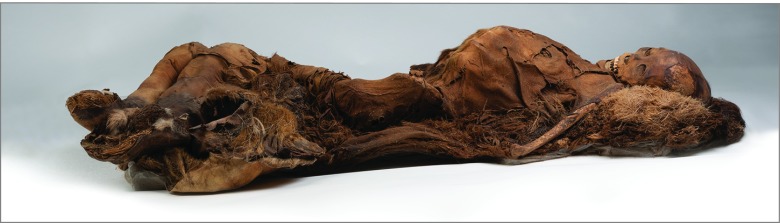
Adult Inuit Mummified Individual Who Was Scanned With Computed Tomography Courtesy of the Peabody Museum of Archeology and Ethnology, Harvard University, PM 29-10-10/61570.0.
